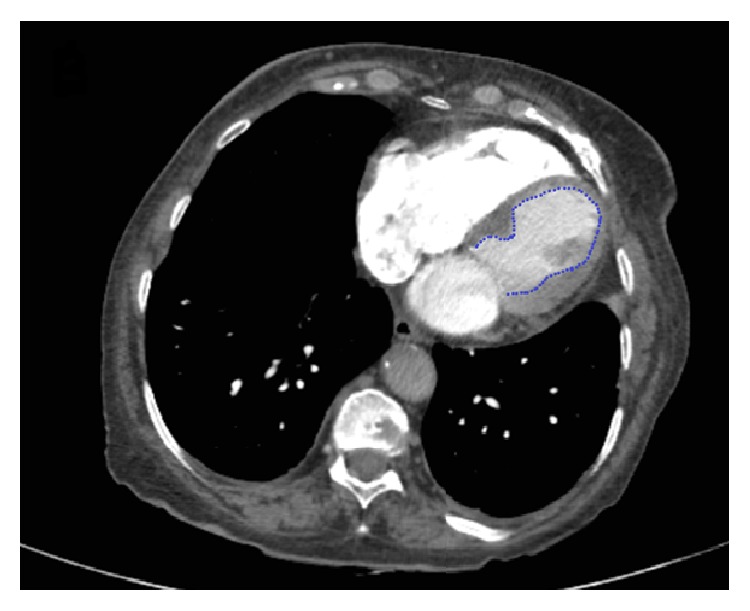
CT angiography showing the integrity of a thin ventricular septum with anterior and apical hypokinesia (apical ballooning pattern) of the left ventricle and also the right ventricle.
